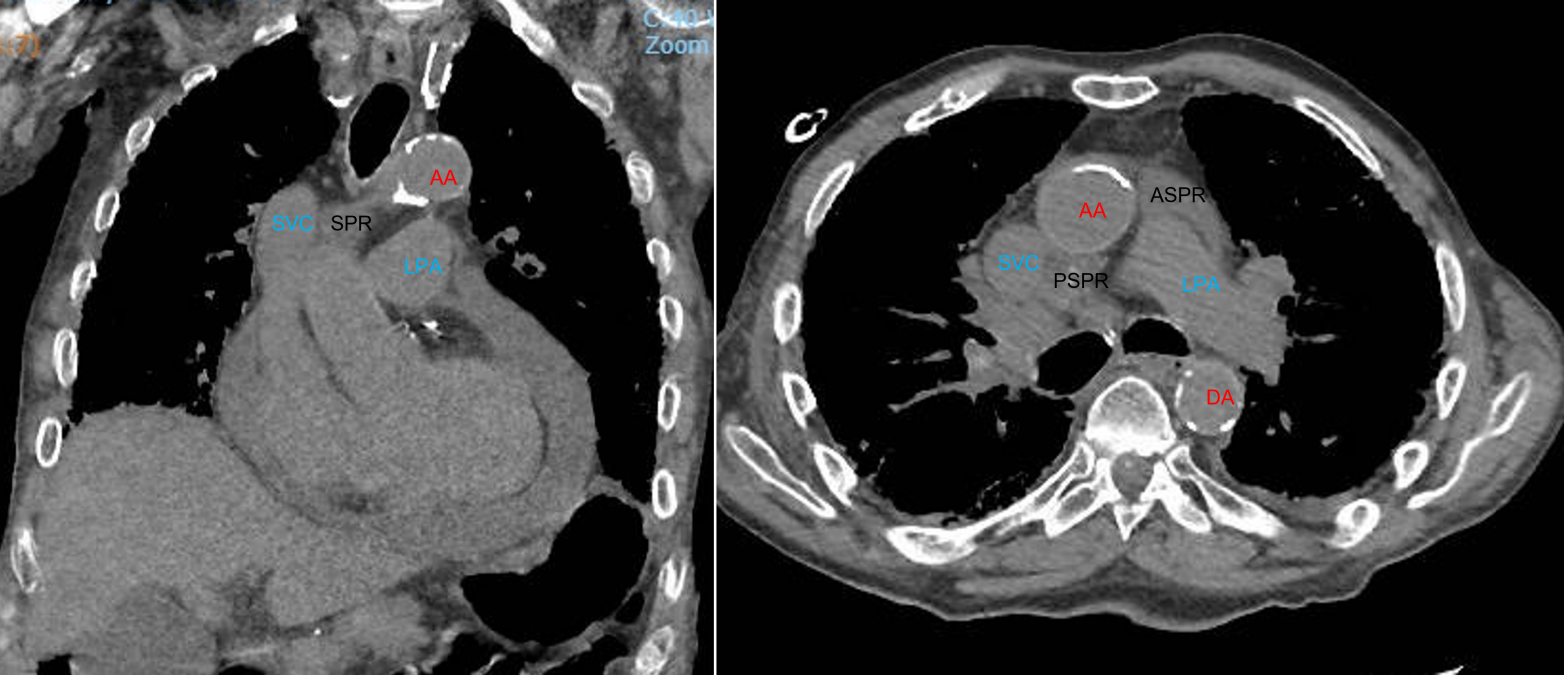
Coronal and axial views of computed tomography chest, demonstrating high riding superior pericardial recess (SPR) both anterior (ASPR) and posterior (PSPR) and its distinction from the ascending aorta (AA), superior vena cava (SVC), left pulmonary artery (LPA) and the descending aorta (DA).
